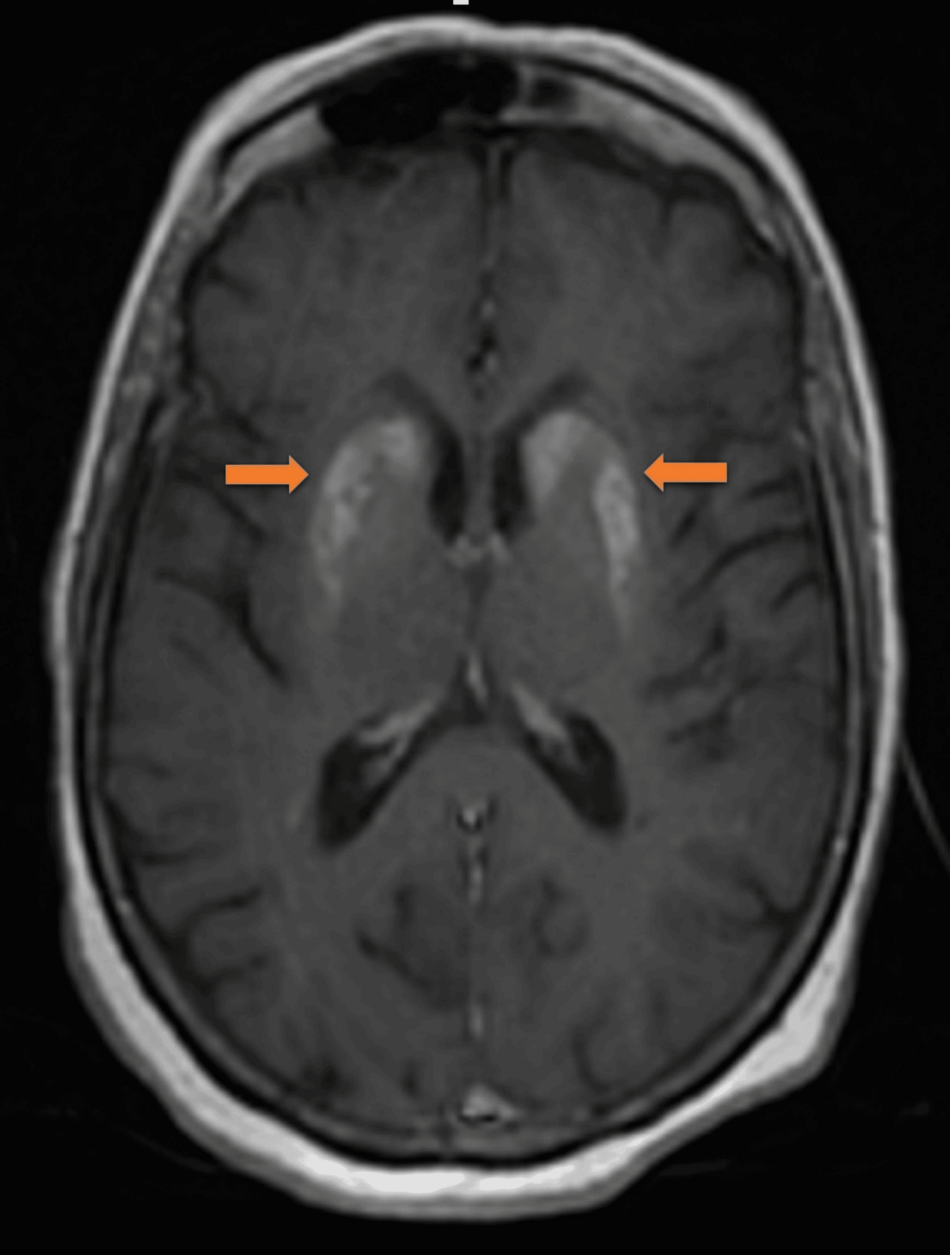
Axial MRI T1 revealing hyperintensities in the striatal region bilaterally MRI: magnetic resonance imaging
